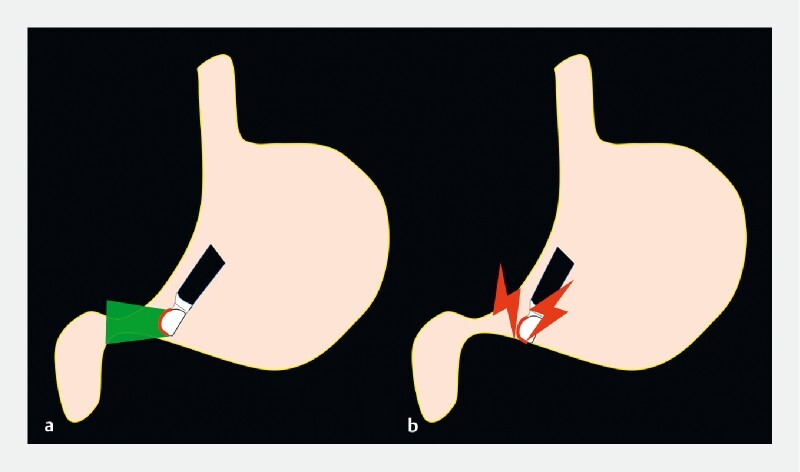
When the endoscopic ultrasound scope is advanced with reference to the endoscopic image (
